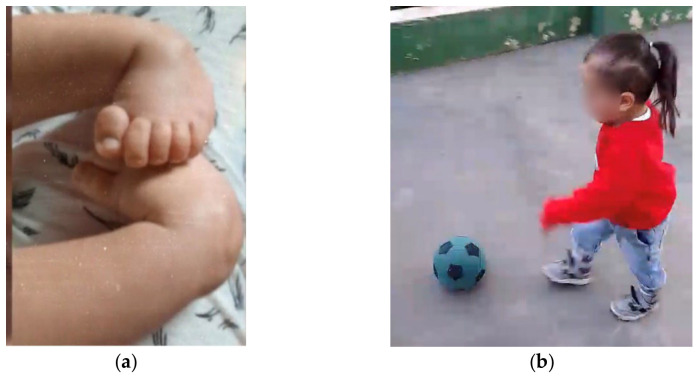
Functional outcome in a child with prenatal clubfoot: (a) Prenatal diagnosis of clubfoot by ultrasound, confirmed at birth; (b) At 3 years of age, the same child demonstrates independent ambulation and age-appropriate motor function, including running and playing.
